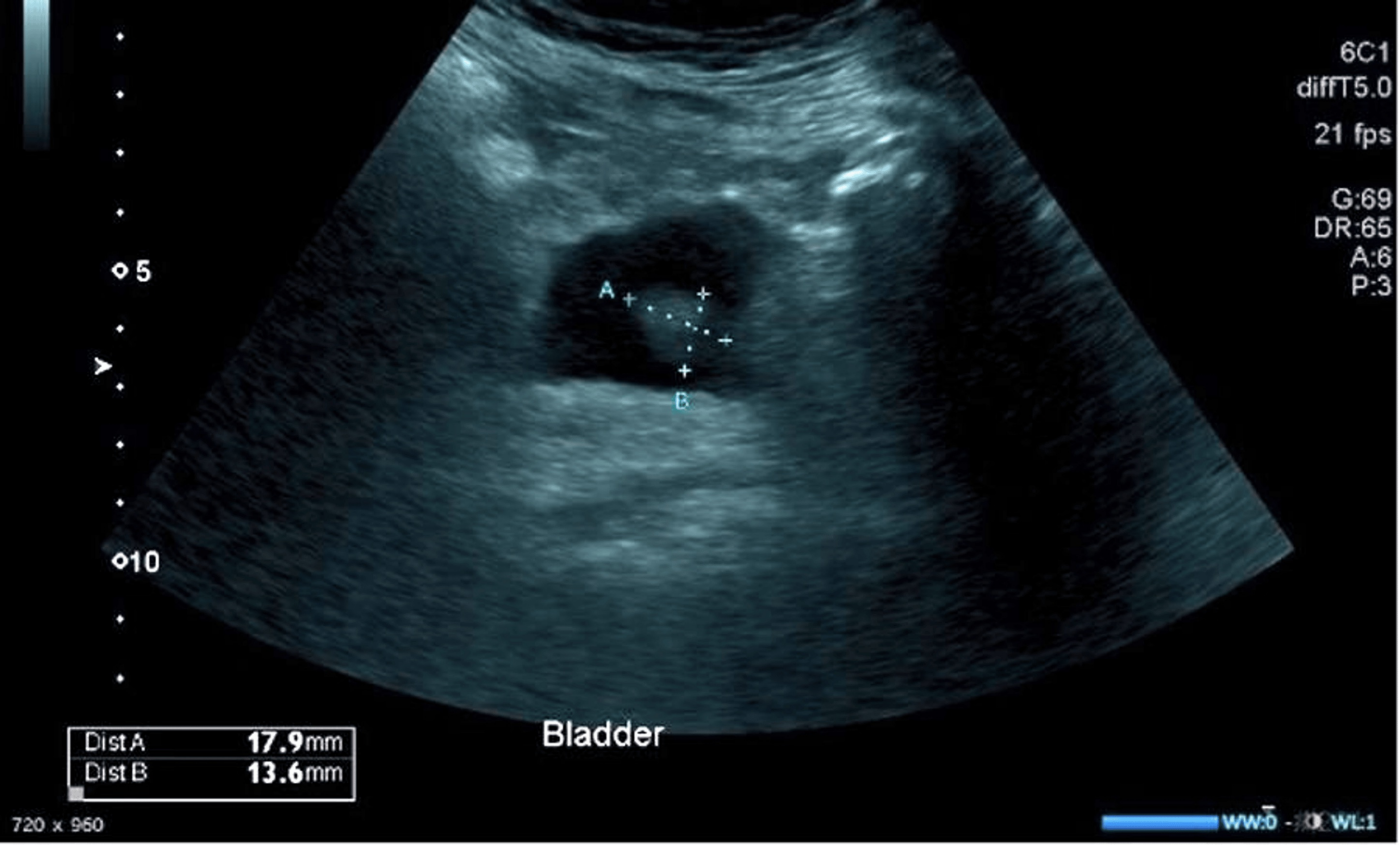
Ultrasound scan of bladder showed an 18x14mm hyperechoic bladder mass arising from the bladder wall.
